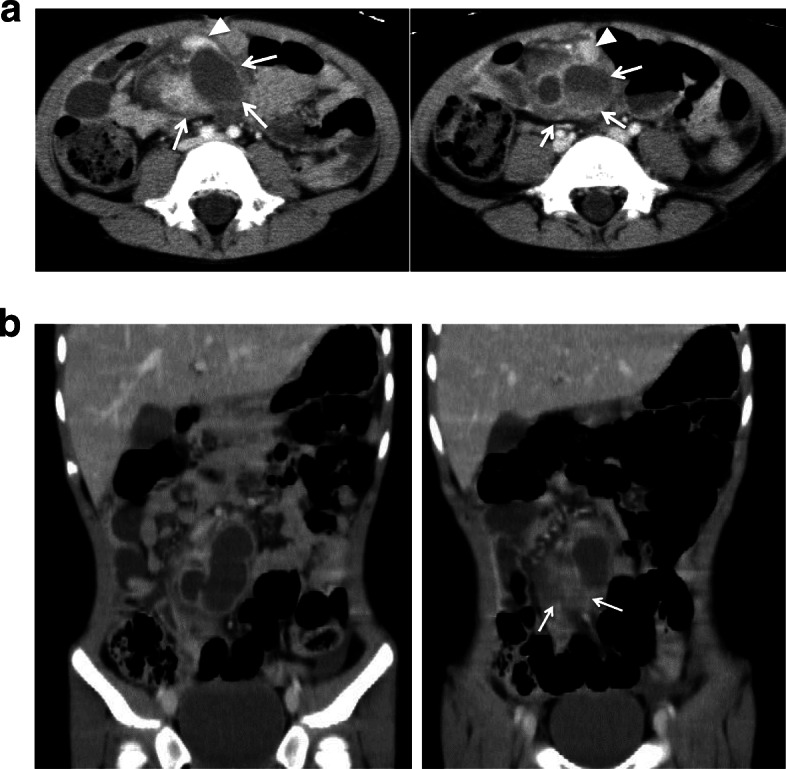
Enhanced CT. a In the ileal mesentery at the level of the umbilicus, a cystic lesion with enhancing wall is seen (arrows). There is a enhancing nodule in the cystic wall (arrow heads). b On coronal plane, the lesion appears to be a tubular structure. The fat density around the lesion is increased suggesting inflammation (arrows)
